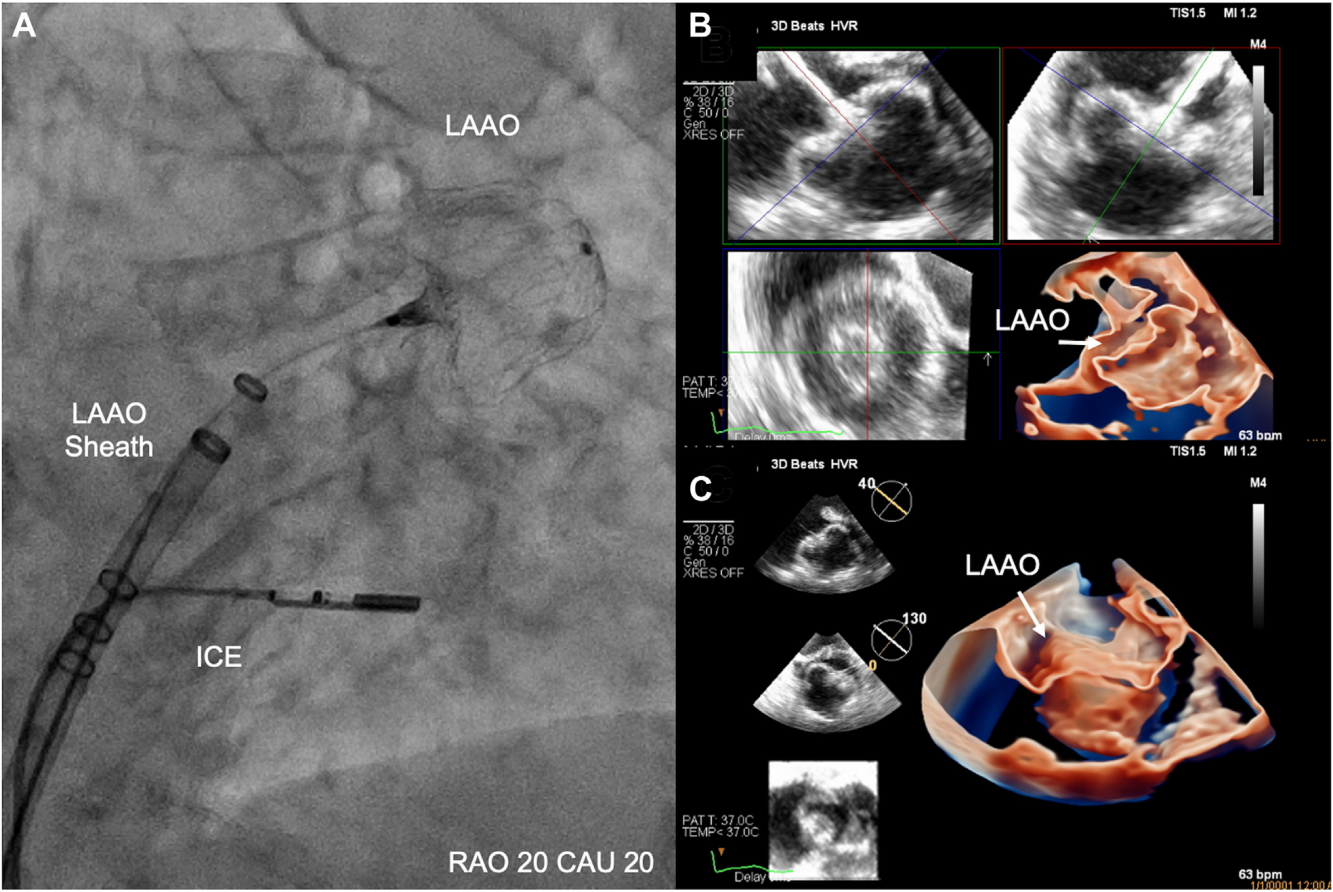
Contrast-Free LAAO Using a Novel 4D-ICE Probe (A) Shows the location of ICE probe on fluoroscopy during deployment. (B, C) Shows the LAA after closure on multiplane 3D imaging from different perspectives. Reprinted with permission from Alkhouli et al. *J Am Coll Cardiol Intv*. 2021:8;14(21):2407-2409. CAU = caudal; ICE = intracardiac echocardiogram; LAAO = left atrial appendage occlusion; RAO = right anterior oblique.
